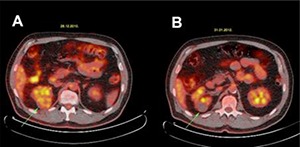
FDG patterns before and after RM-CA in patient number 7. Before treatment (A), the renal tumor in the right kidney shows a low FDG uptake at ^18^F-FDG PET-CT. The PET-CT scan after treatment (B) shows the same tumor without signs of the existence of vital tissue. Green arrows indicate the tumor before (A) and after (B) cryoablation.
